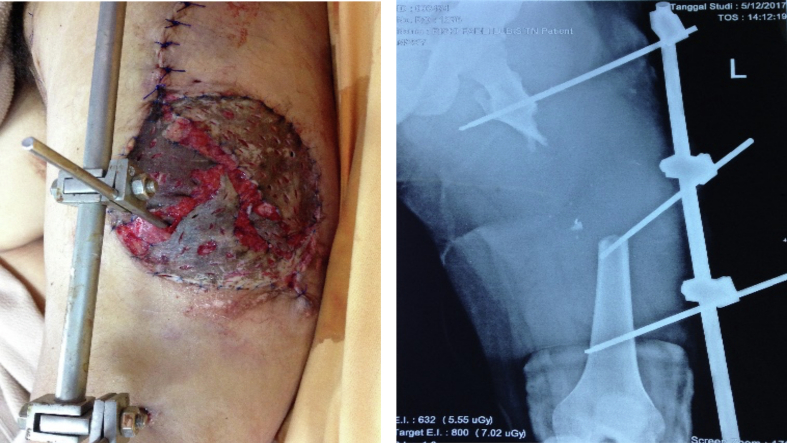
A 9 x 7 cm soft tissue defect located on the left thigh patient and the left femur x-ray showed a displaced fracture of the left femur that had been immobilized by external fixation.
